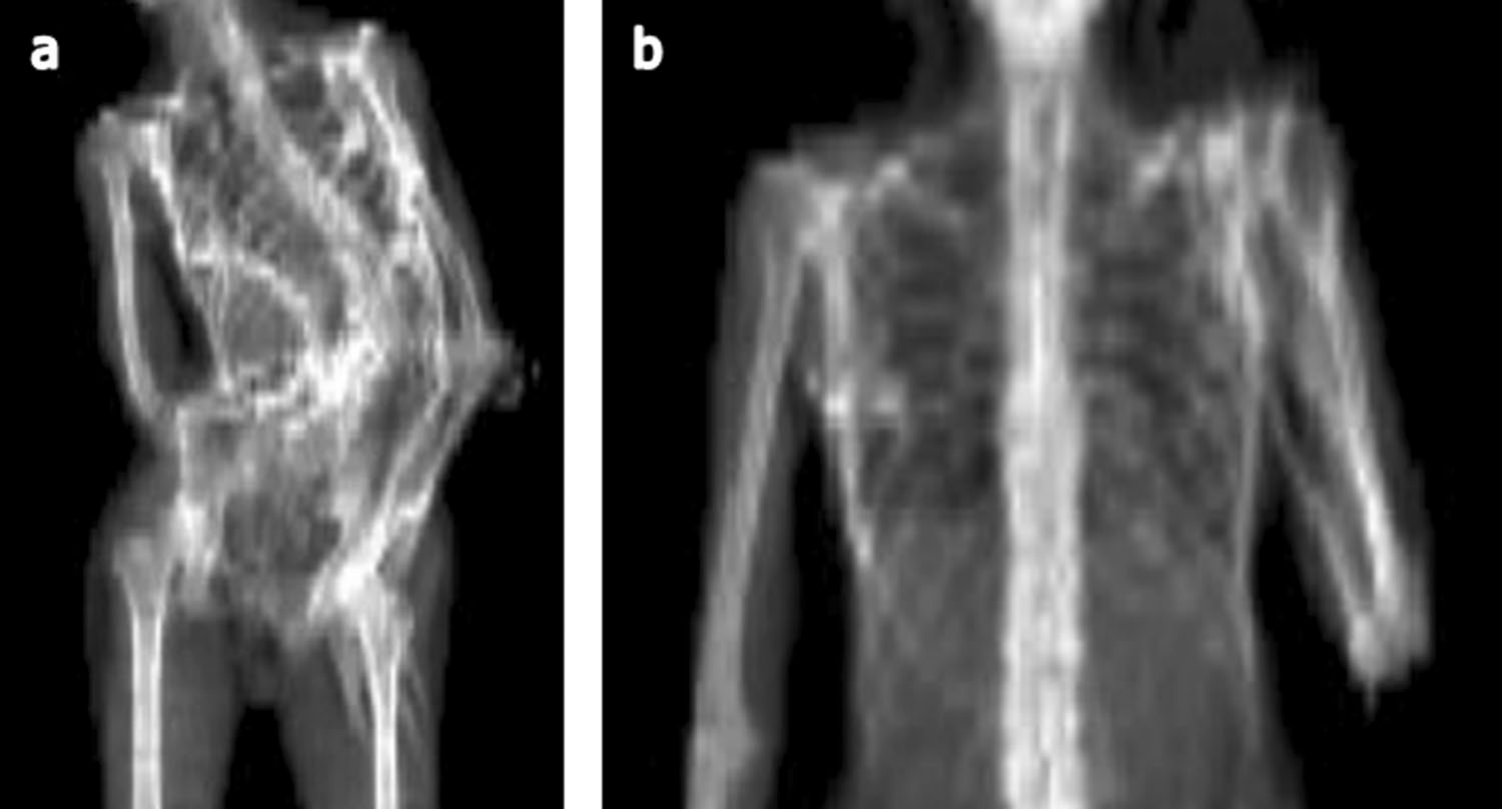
Overlapping anatomy precludes HO evaluation using a DXA or b CT scout scans. Image a was taken from an 18-year-old male, with a Baseline CAJIS score of 14 at enrollment. Image b was taken from a 34-year-old female, with a Baseline CAJIS score of 12 at enrollment. *CT* computed tomography; *DXA* dual energy X-ray absorptiometry, *HO* heterotopic ossification
